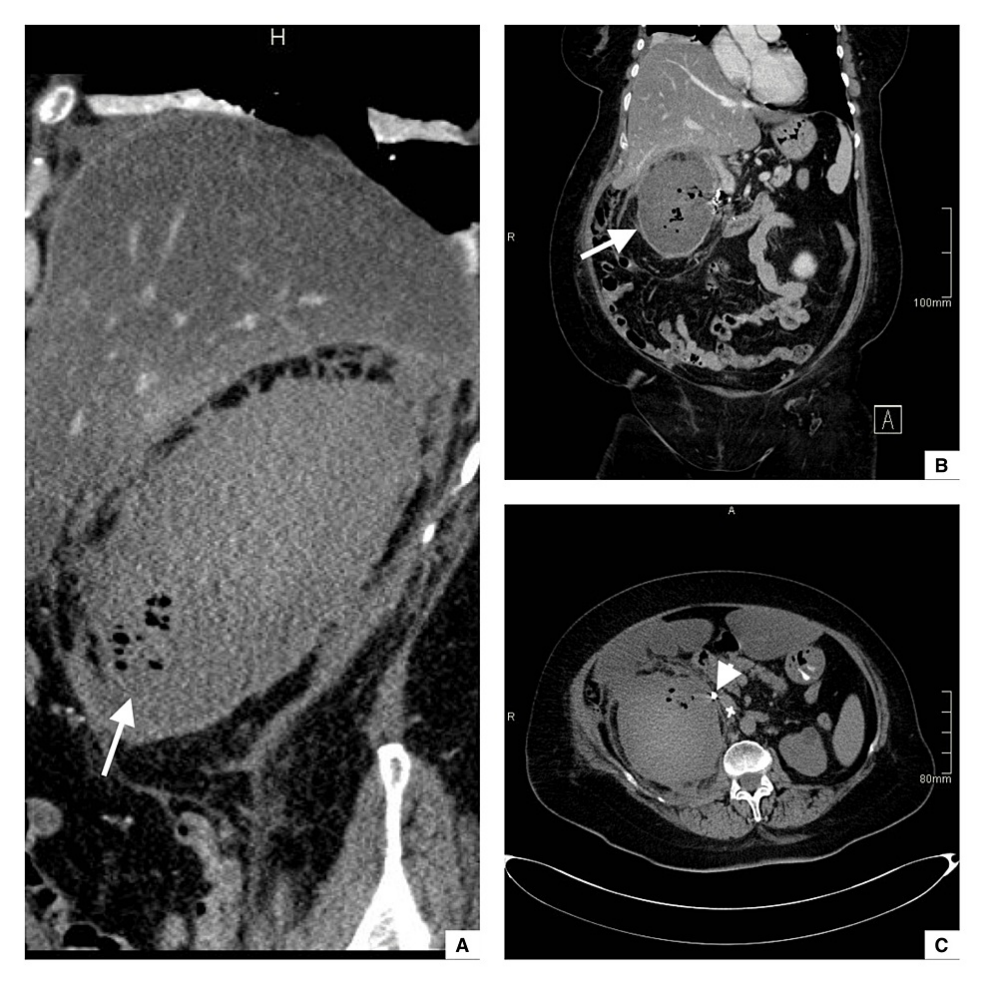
Enhanced sagittal and coronal CT scan A and B: An infected hematoma with air pockets (white arrows), C: Coil embolization (arrowhead)
